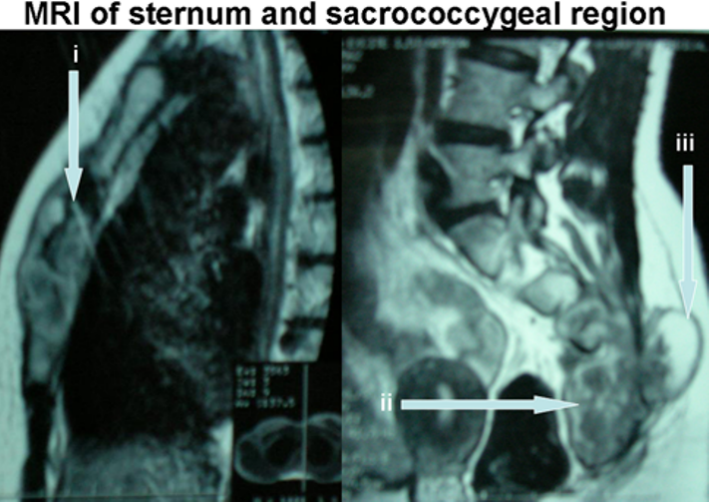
Magnetic resonance imaging of the sternal and sacrococcygeal regions. *i* Destructive lesion in the sternum, *ii* destructive lesion in the coccyx with an abscess, *iii* abscess extending into the left gluteal region
